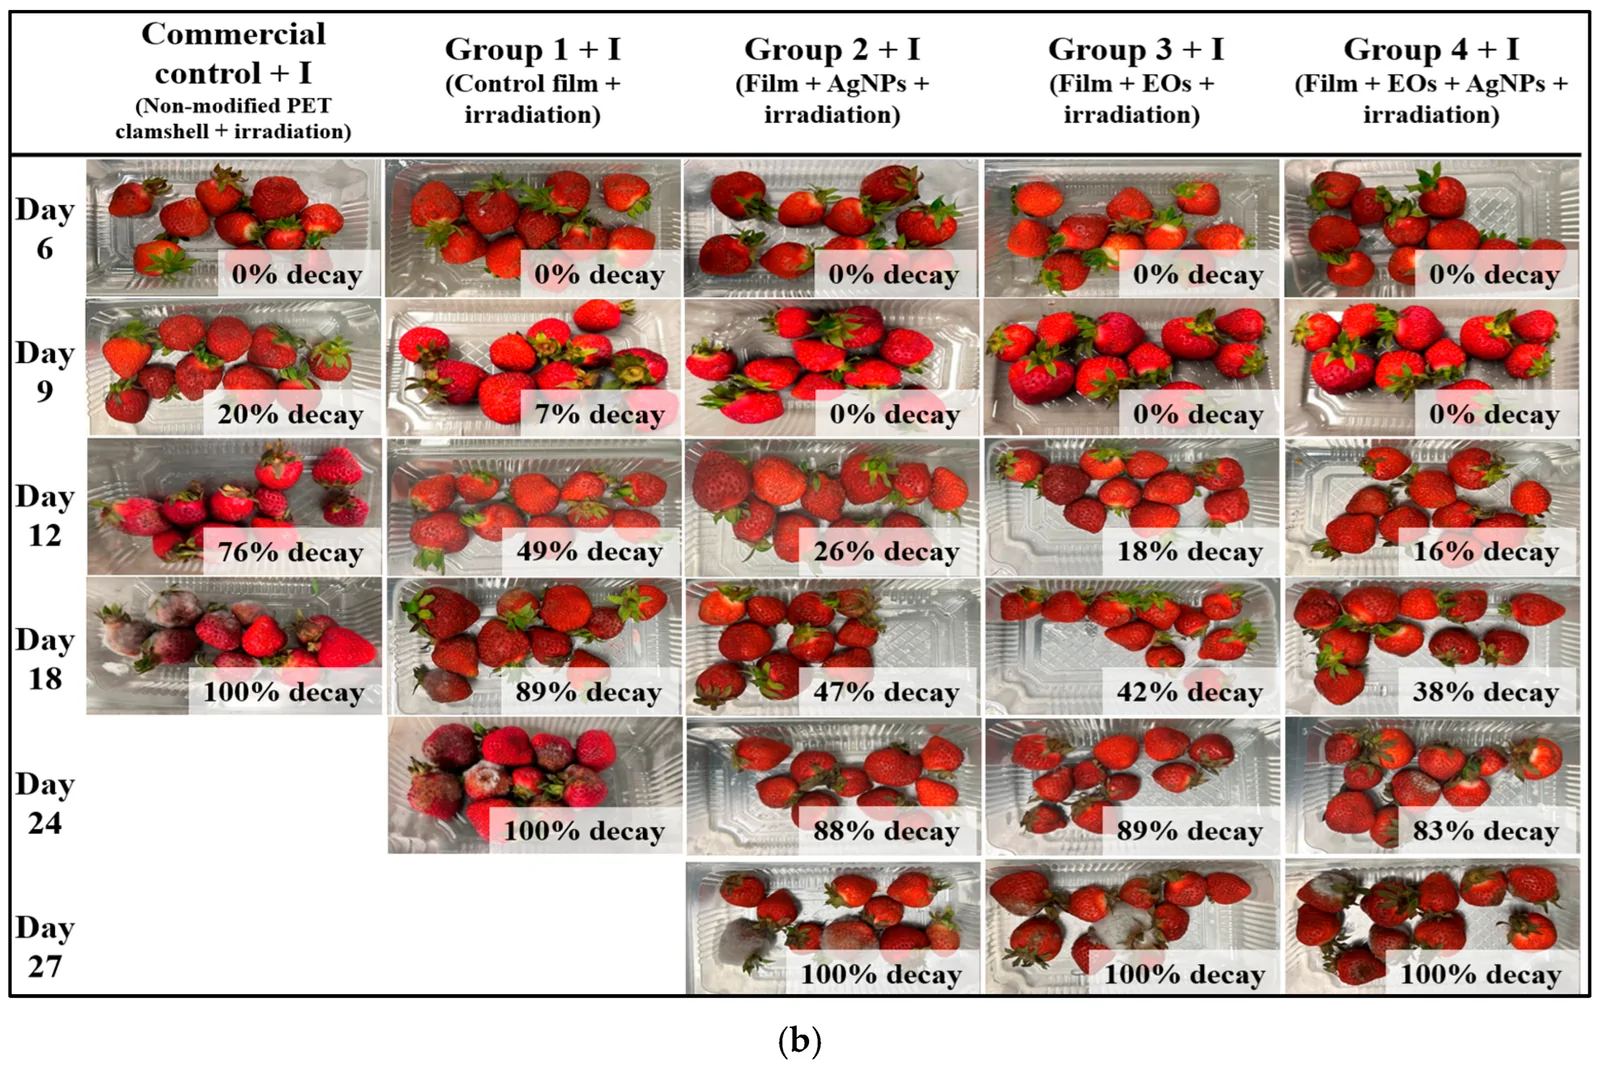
Decay rate and fruit appearance in packaging (a) and in packaging combined with L-E X-ray irradiation (b).
